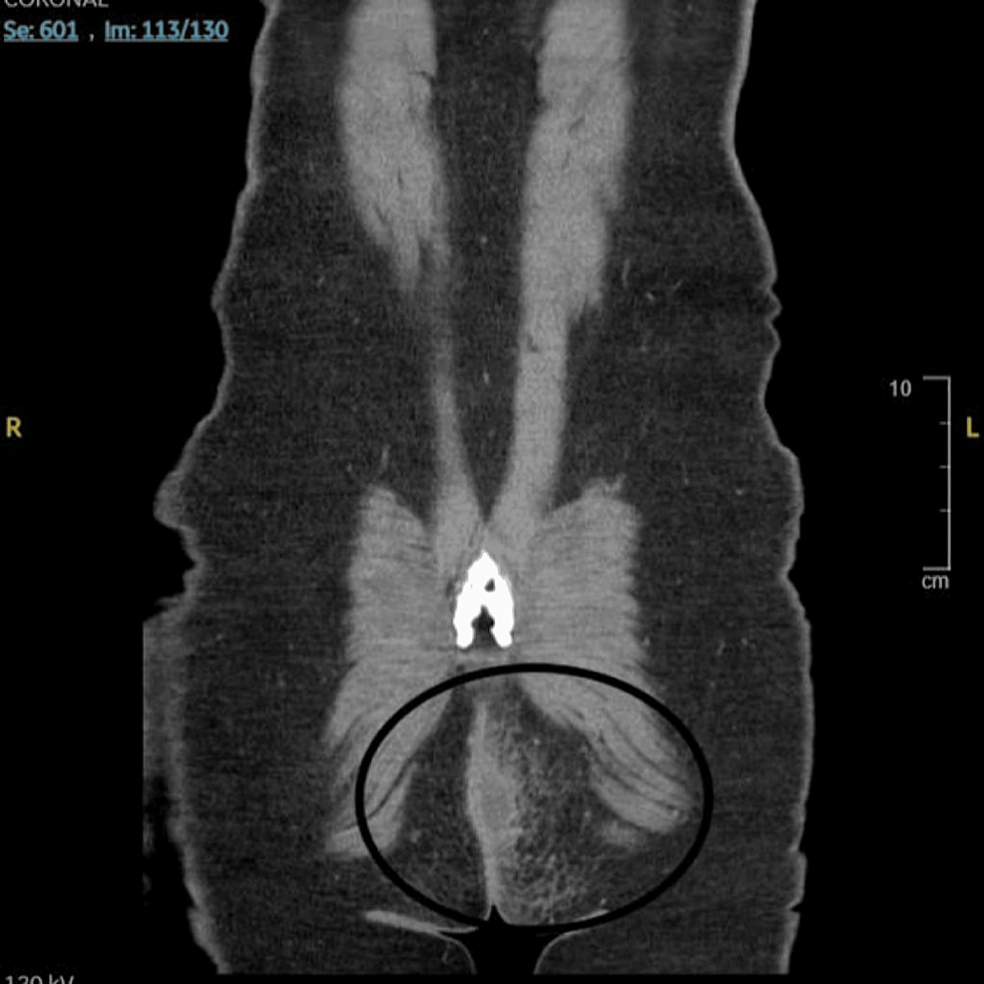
Coronal cross-sectional CT showing left perianal abscess
